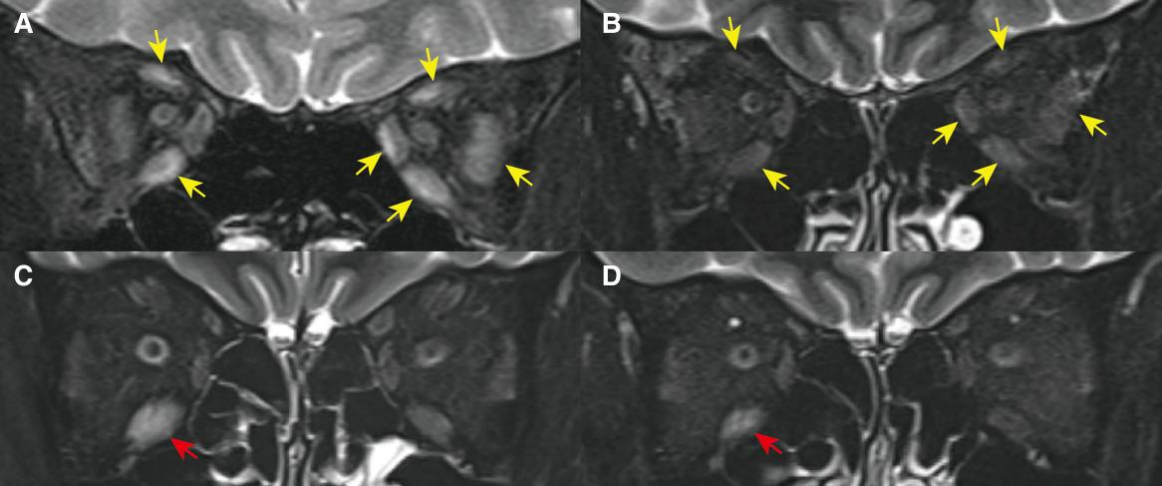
Short tau inversion recovery images of orbital magnetic resonance imaging during treatment for Graves orbitopathy. Enlarged extraocular muscles with high signal intensities (A, yellow arrows) improved after treatment with steroid pulse therapy and ocular radiotherapy (B, yellow arrows) on first admission. Enlargement of the right inferior rectus muscle with high signal intensities after SARS-CoV-2 infection (C, a red arrow), which improved after the combination therapy (D, a red arrow) on second admission.
